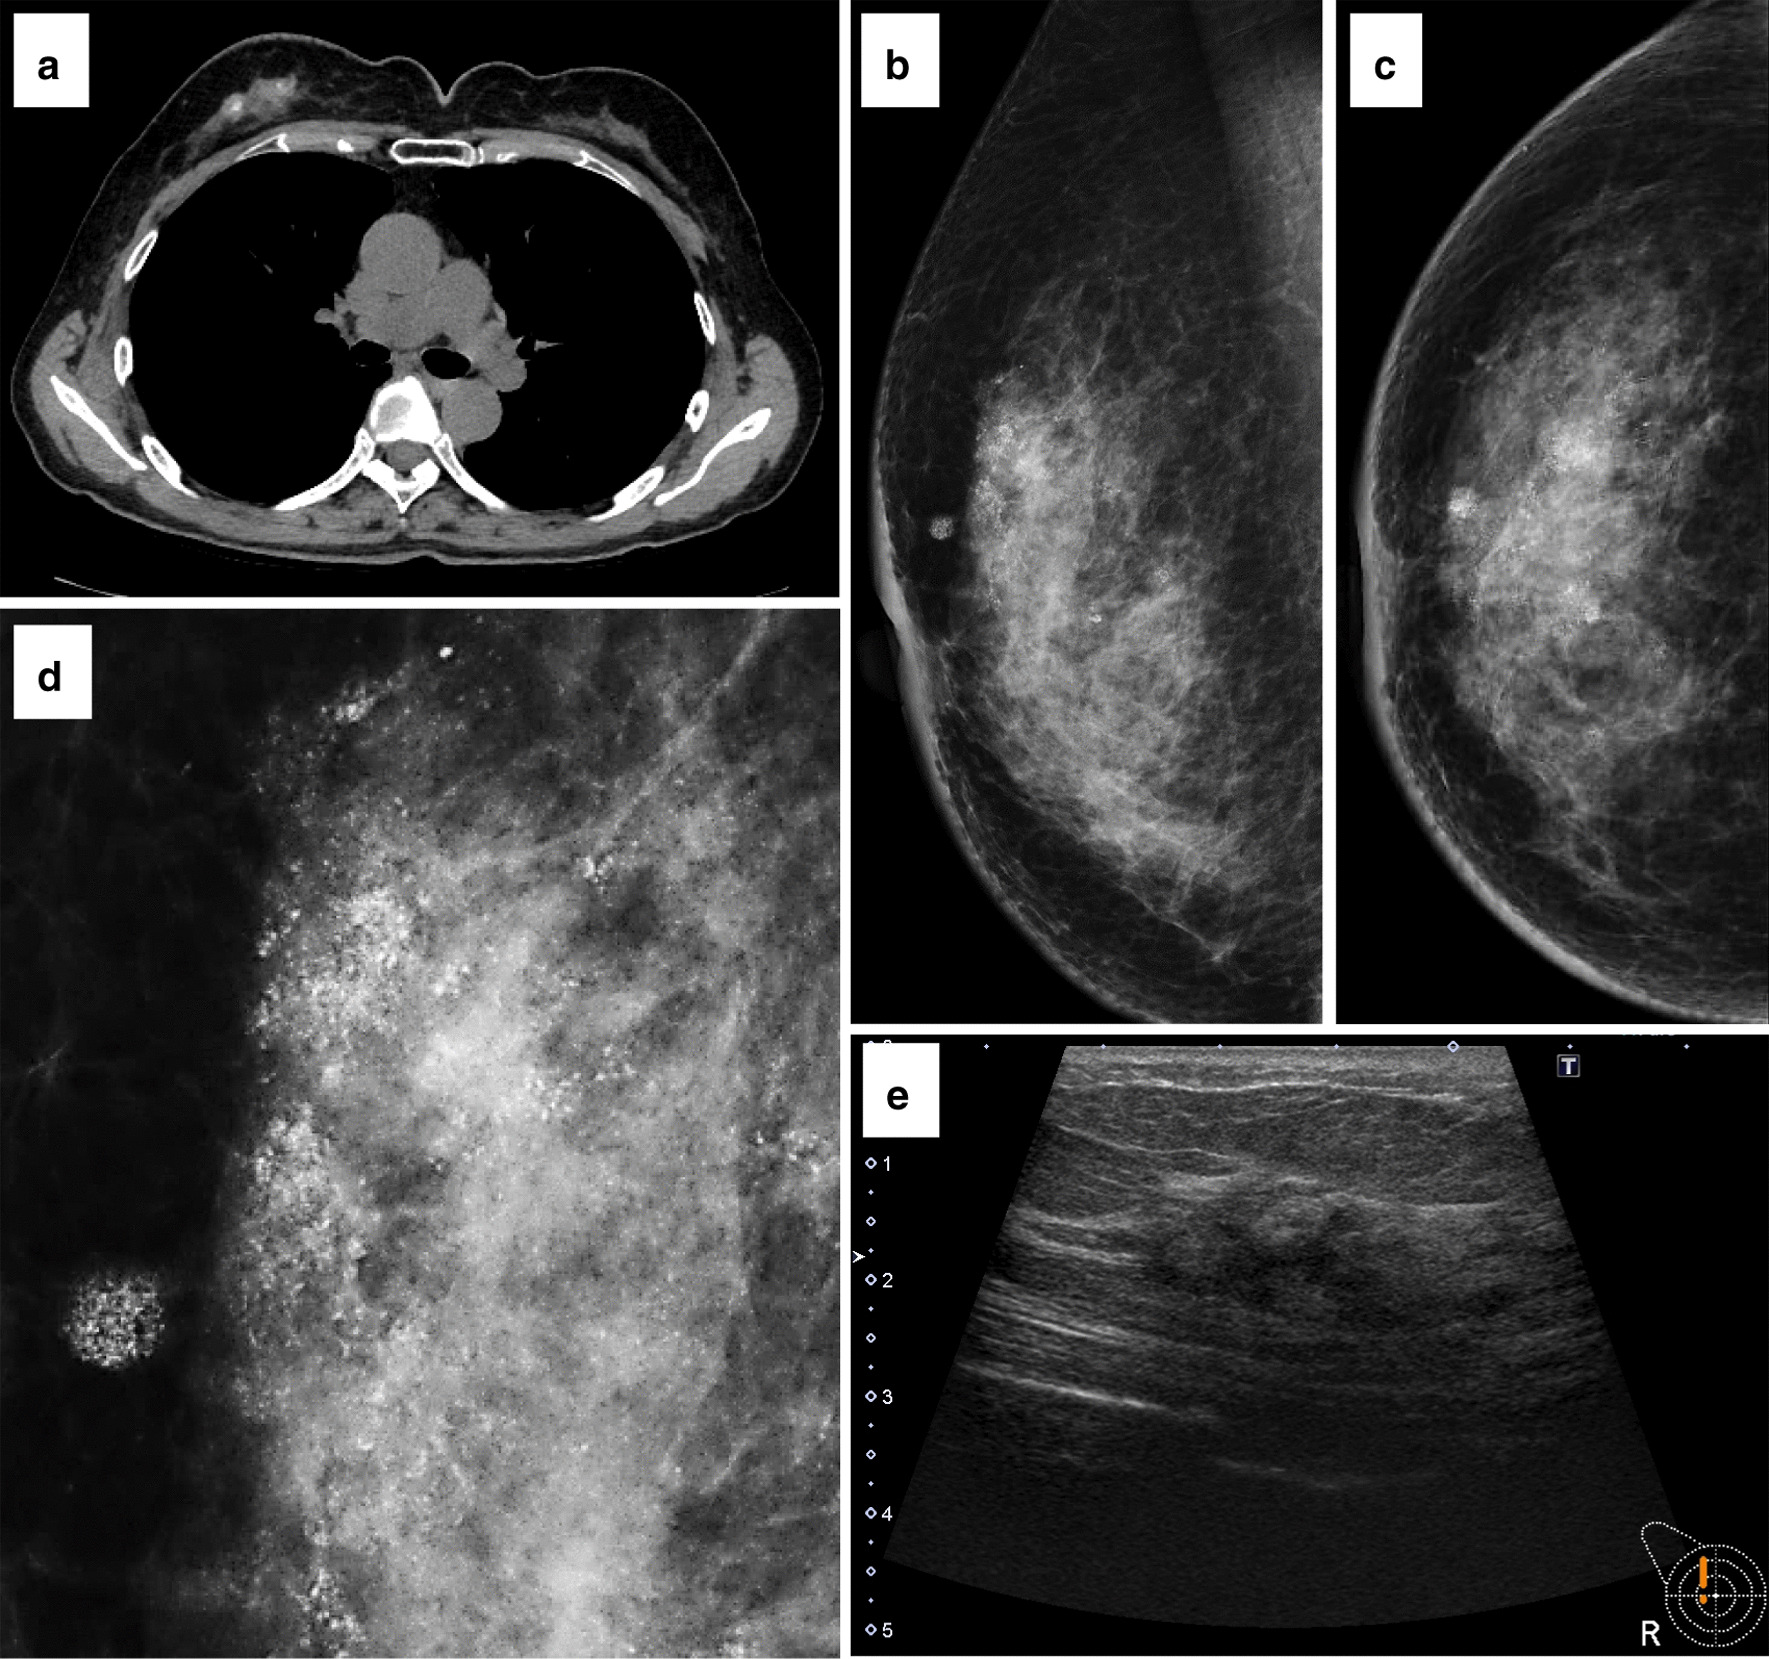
Radiological images of the metastatic ovarian serous carcinoma in the breast. Plain chest computed tomography (a); mediolateral-oblique and craniocaudal views of the mammography (b, c) and magnified image of the mediolateral-oblique view (d); and ultrasonography (e)
